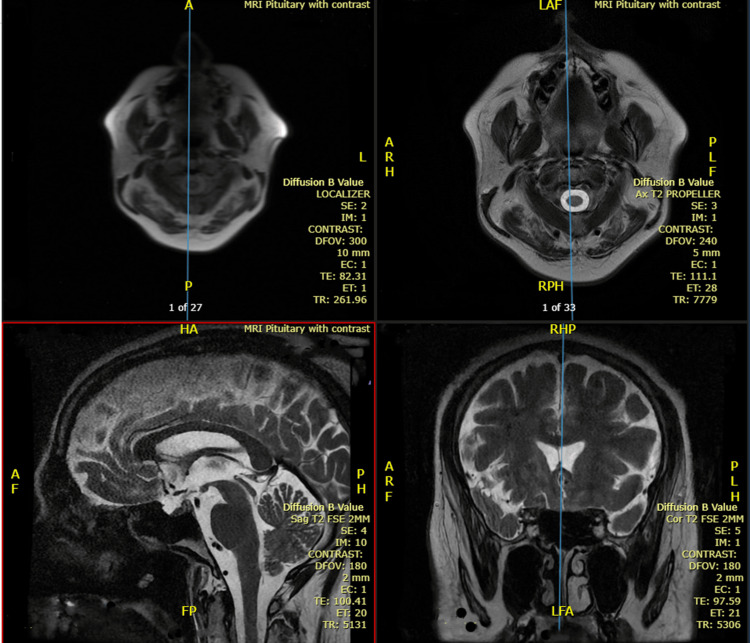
MRI of the brain in sagittal and coronal views showing normal brain parenchyma
